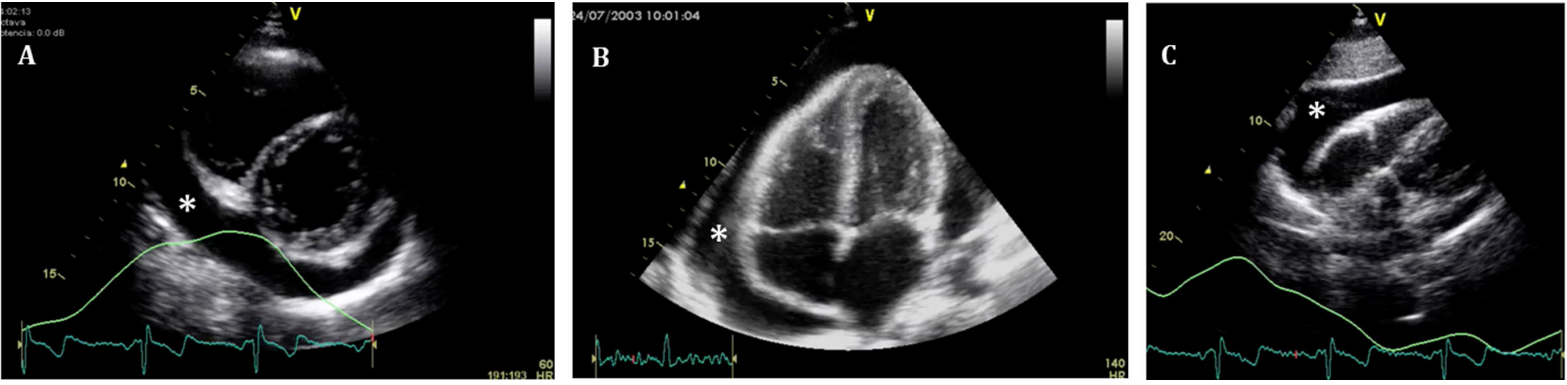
Standard echocardiographic views to assess a pericardial effusion (*). (A) Parasternal short axis; (B) four-chamber view; and (C) subcostal view.
